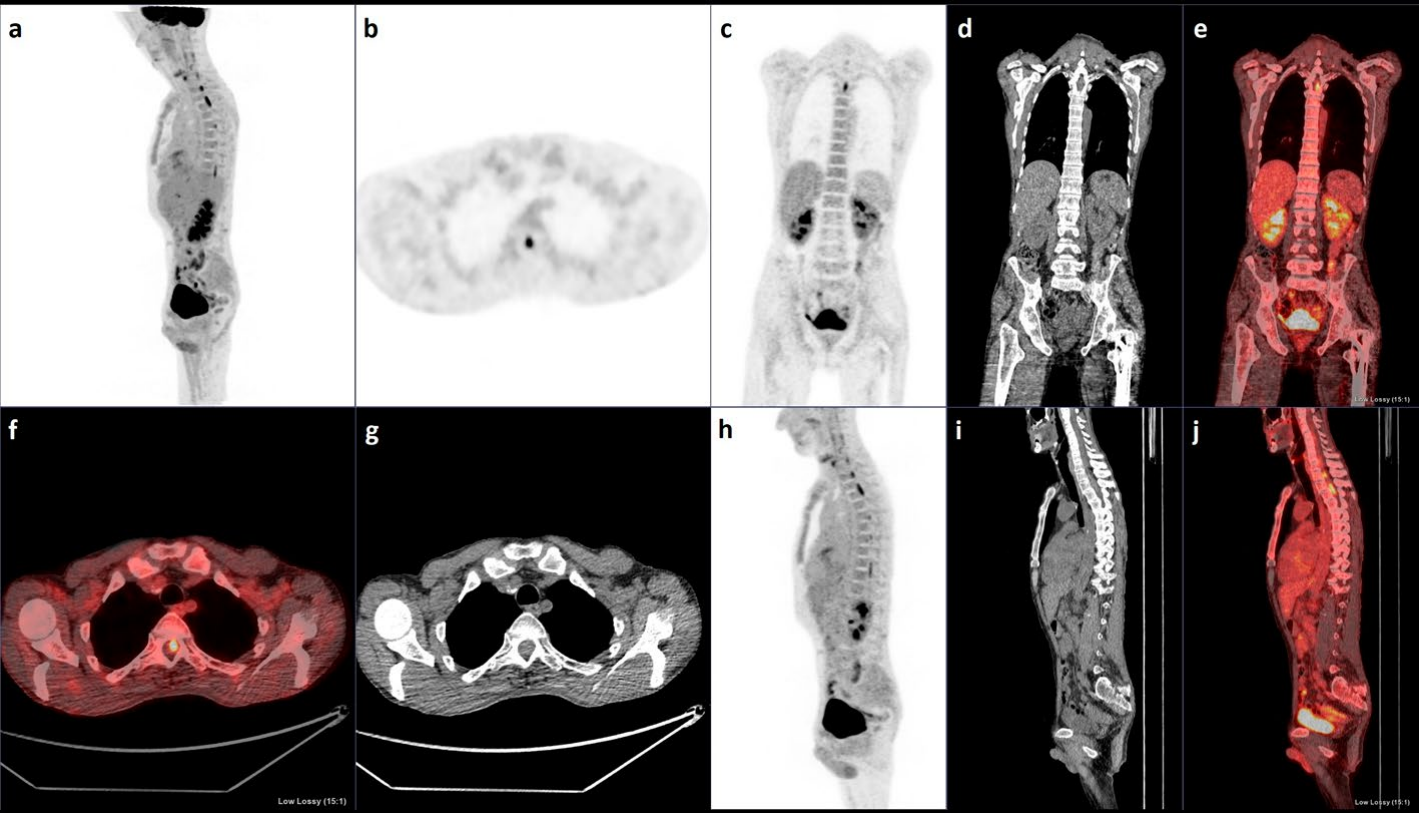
Sagittal MIP image (a) from whole-body ^18^F-FDG-PET/CT demonstrates intense multifocal segmental uptake in the cervical and thoracic spine (SUVmax 9.6). Axial (b, f, g), coronal (c, d, e) and sagittal (h, i, j) images confirm the intramedullary location of the lesions
